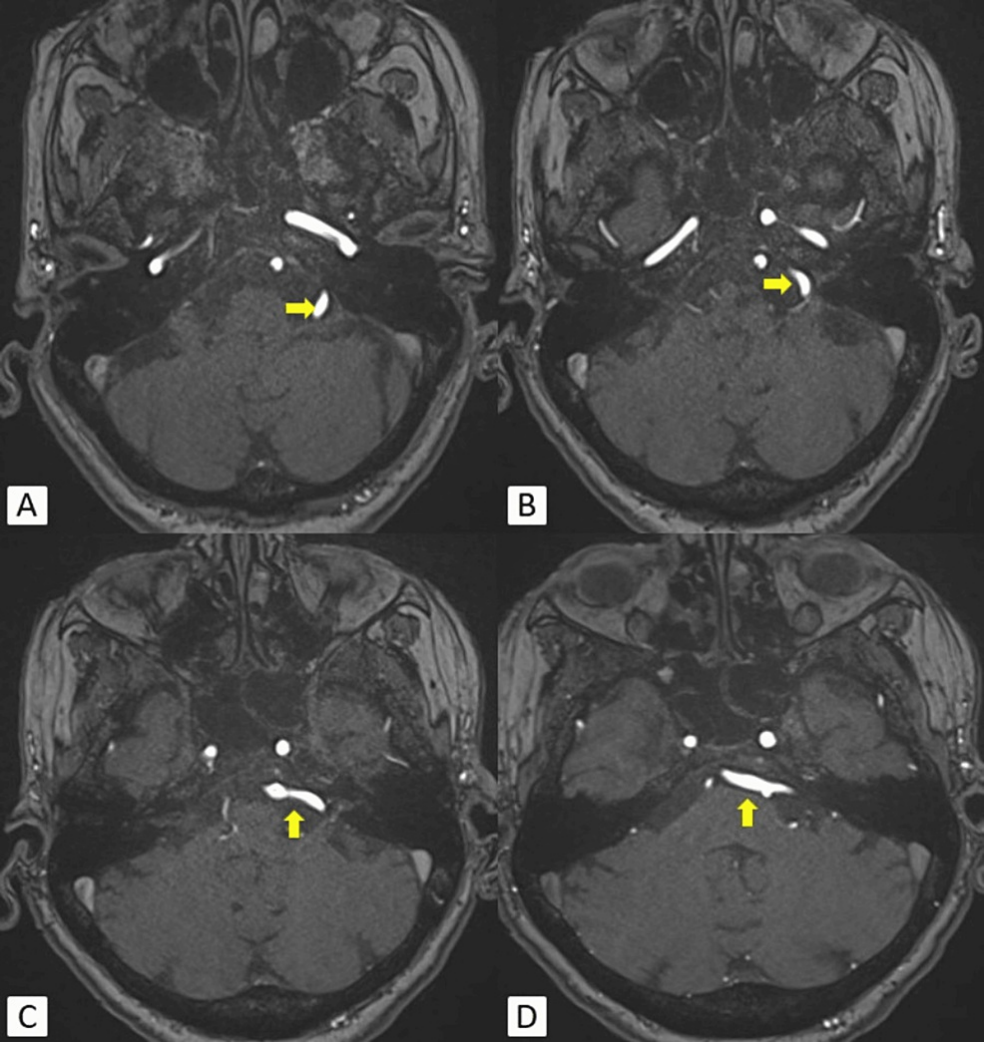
(A-D) 3D TOF MRI of the brain angiography showing dolichoectasia of the left vertebral artery (yellow arrows) extending in the left CP angle cistern causing mild compression over the left VII-VIII nerve complex. TOF: time of flight; CP: cerebellopontine; MRI: magnetic resonance imaging
